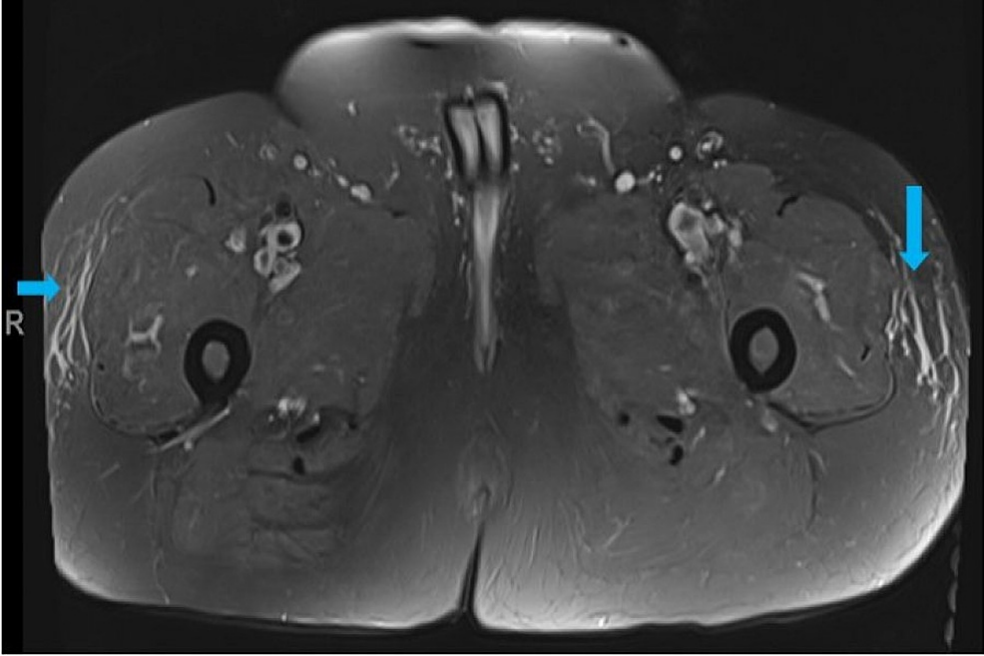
MRI-left quadriceps: axial view There is diffuse oedema in the subcutaneous fat tissue on the anterolateral sides of both thighs (blue arrows).
